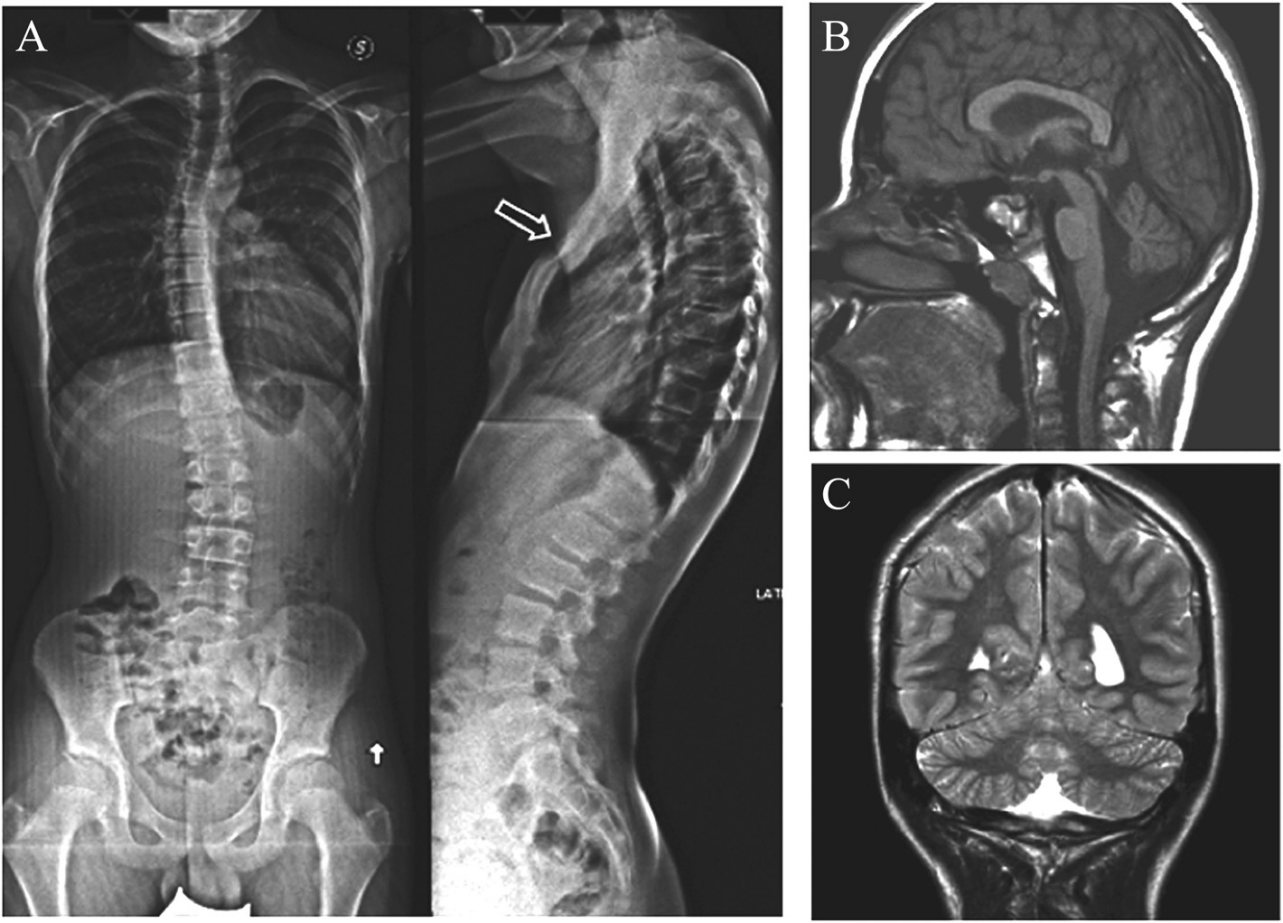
X-ray and MRI analyses of the patient. A) Standing X-ray films of full-length spine. Front view showing thoracolumbar scoliosis and side view demonstrating pectus excavatum (open arrow). No associated vertebral abnormalities are present. B, C) Brain MRI.) Midsagittal T1-weighted image demonstrating moderate vermis hypoplasia (B). Coronal T2-weighted image showing normal cerebellar hemispheres (C).
